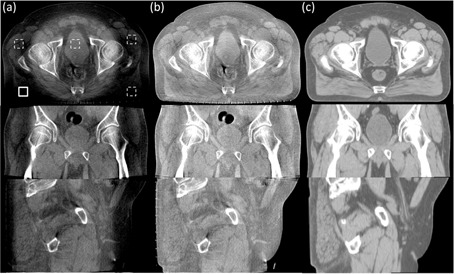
The axial, coronal, and sagittal comparison views of the prostate patient images. Display window: [−250 250] HU: (a) CBCT without correction; (b) CBCT with the proposed shading correction; (c) registered pCT. In the selected uniform soft‐tissue ROI (marked with a solid white square in (a)), the average CT numbers from (a) to (c) are −252 HU, 10 HU, and 52 HU, respectively. The SNUs calculated on the selected five ROIs (marked with solid and dashed white squares in (a)) are 21.2%, 3.2%, and 1.1%, respectively.
